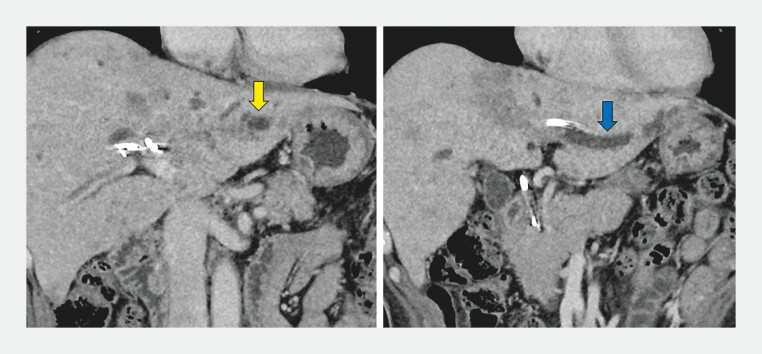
CT image demonstrating cholangitis in the undrained lateral segment despite placement of four stents for malignant hilar biliary obstruction. The B2 (yellow arrow) and B3 (blue arrow) ducts are isolated and noncommunicating.
